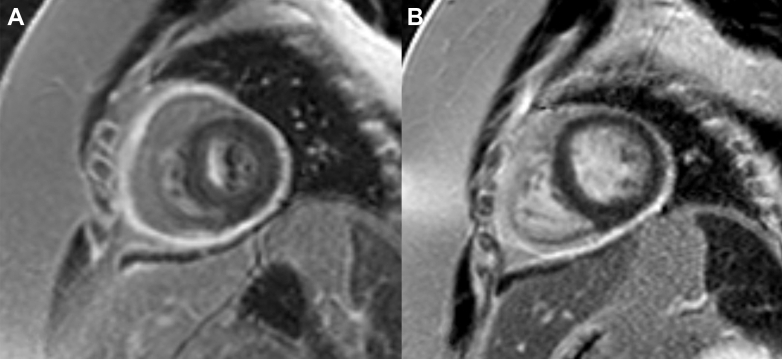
Cardiac Magnetic Resonance Imaging (A) Cardiac magnetic resonance (CMR) phase sensitive inversion recovery (PSIR) late gadolinium enhancement (LGE) sequence demonstrating increased thickness of the pericardium, and severe circumferential pericardial enhancement and increased thickness. (B) PSIR LGE sequence obtained after pericardiectomy, demonstrating reduced burden of residual patchy LGE in the inferior pericardium.
